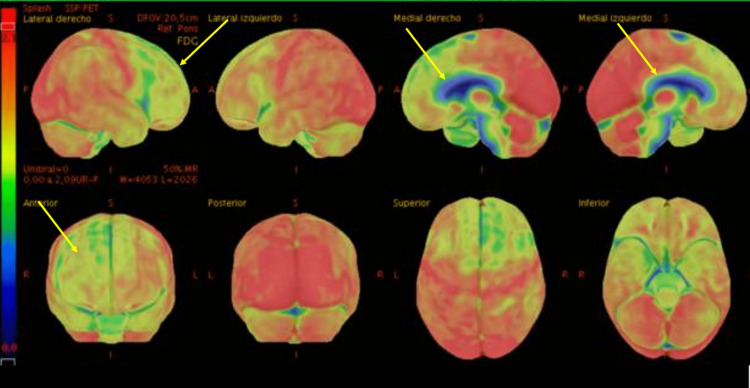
Brain 18F-FDG PET scan that shows moderate to severe bilateral prefrontal cortex hypometabolism that extends to the orbitofrontal cortex and the insular region. 18F-FDG PET: 18F-fluorodeoxyglucose positron emission tomography
